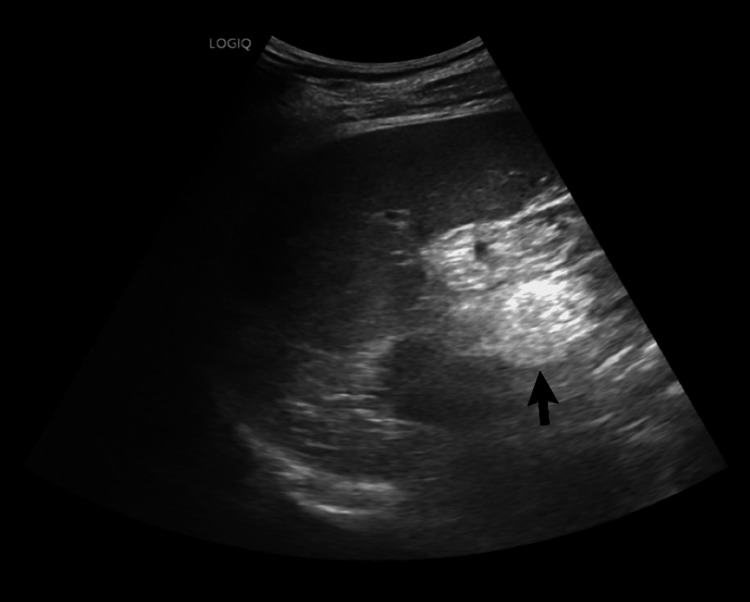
Ultrasound of the abdomen demonstrating hydroureteronephrosis. A routine grayscale ultrasound of the right upper quadrant demonstrating a dilated pelvicalyceal system (arrow) with marked parenchymal thinning, consistent with moderate-to-severe right-sided obstructive hydroureteronephrosis.
